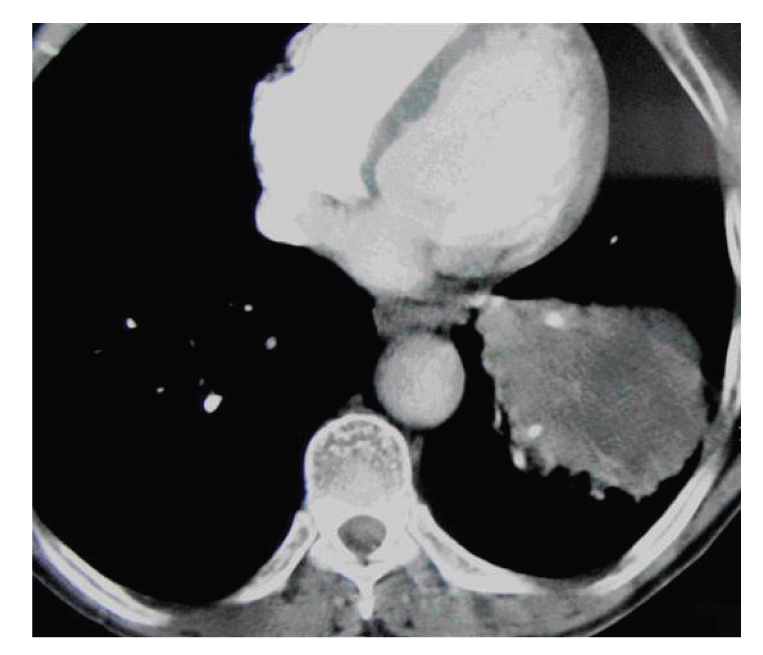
Thoracic computed tomography (a hypodense mass lesion at the left pulmonary lobe).
